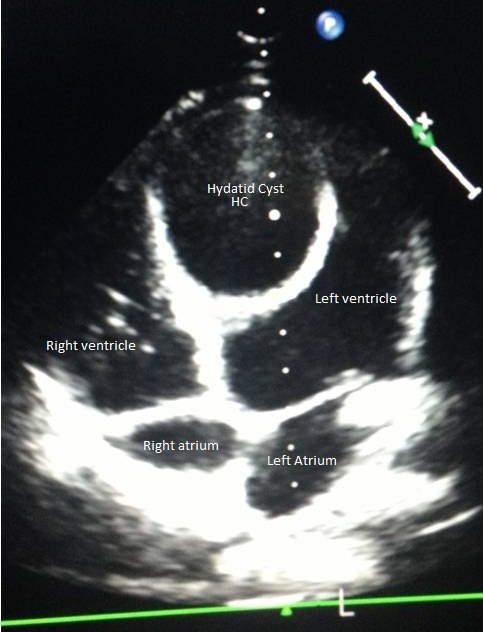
TTE: echocardiographic appearance of left ventricular hydatid cyst
